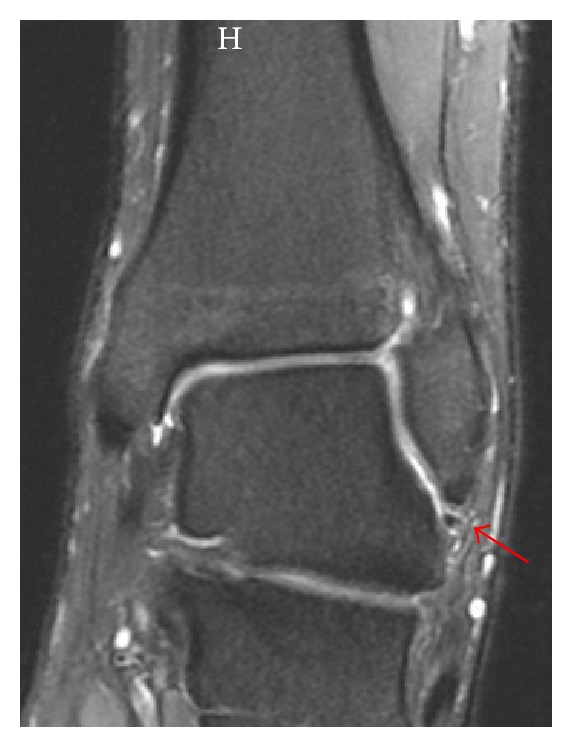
Coronal proton density fat saturated MR image showing an ATFL tear (arrow).
